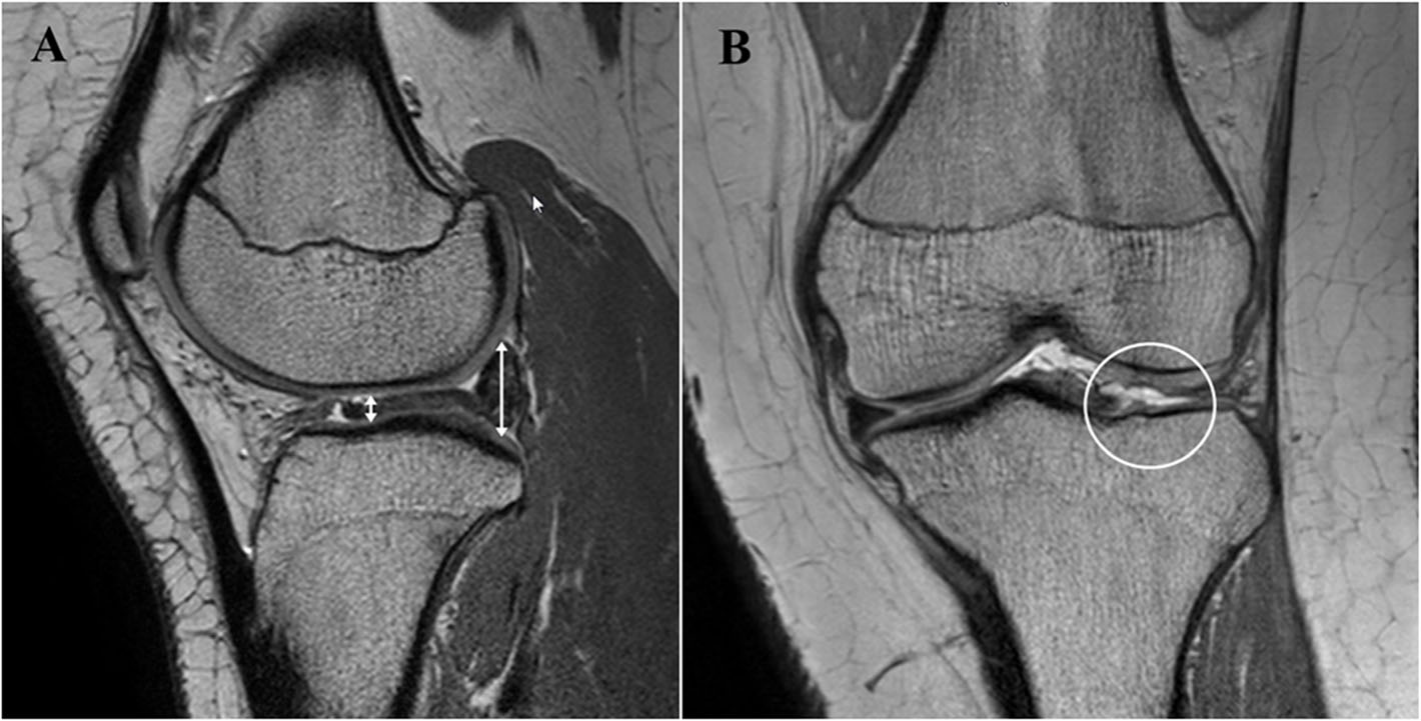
Displacement of discoid lateral meniscus on MRI. (A) the difference of thickness between the anterior and posterior horn is more than 2-fold increase in sagittal image (*two sided arrows*: thickness of meniscus). (B) the signal loss is observed on anterior side of coronal image (*empty circle*: detached side of meniscus)
